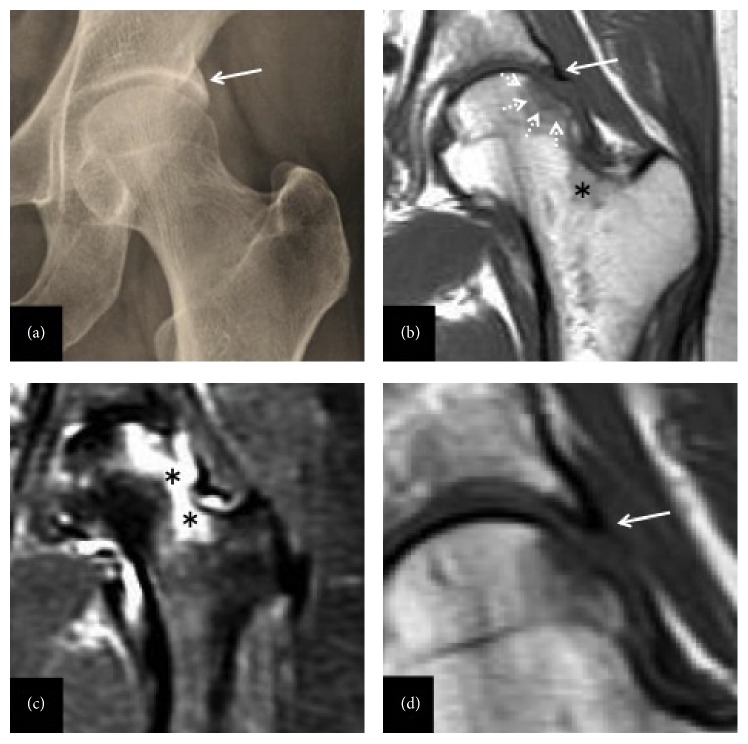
Initial radiograph and magnetic resonance imaging (MRI) of the left hip. (a) Anteroposterior view of the left hip shows acetabular overcoverage due to extended lateralization of the acetabular margin (white arrow). (b) T1-weighted image shows bone marrow edema with diffuse low intensity expanding from the lateral aspect of the femoral head to the intertrochanteric area. An irregular, serpentine, low intensity band (white dotted arrow) is present in the subchondral area. (c) Short-tau inversion recovery (STIR) image shows high signal intensity corresponding to the area of low intensity in (b). Black asterisks indicate the bone marrow edema of the femoral head and intertrochanteric area. (d) No apparent labral tear at the extended rim was observed but the labrum was relatively small and thin on the T1-weighted image (white arrow).
